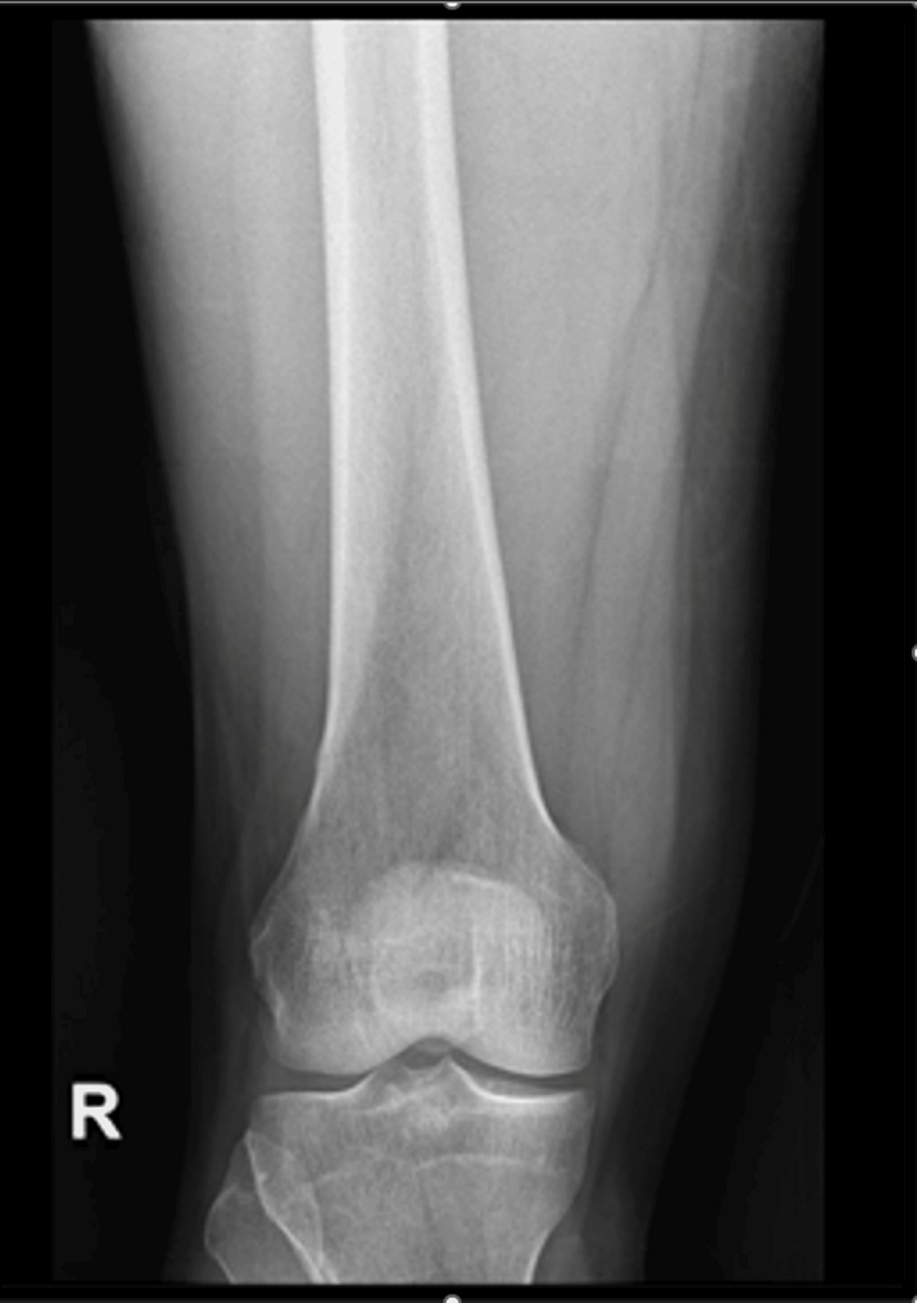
Anterior-posterior X-ray view Normal X-ray of the femur bone
